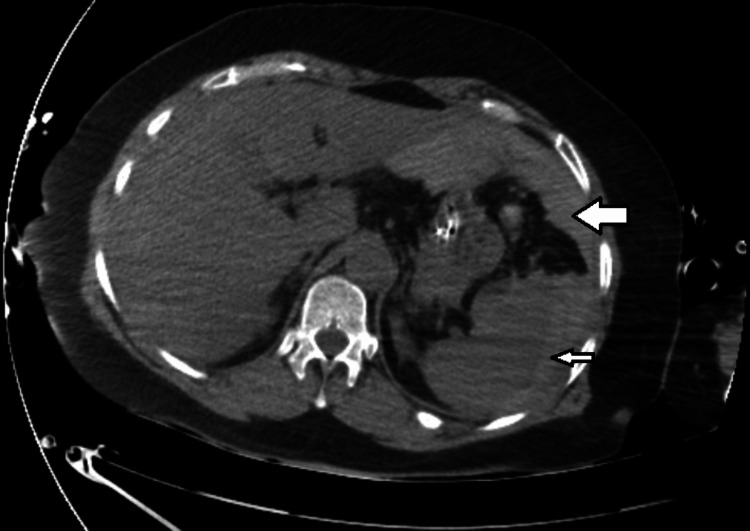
Computed tomographic scan of the abdomen on the 4th day in the intensive care unit. Hemoperitoneum (big arrow) due to splenic laceration (small arrow)
